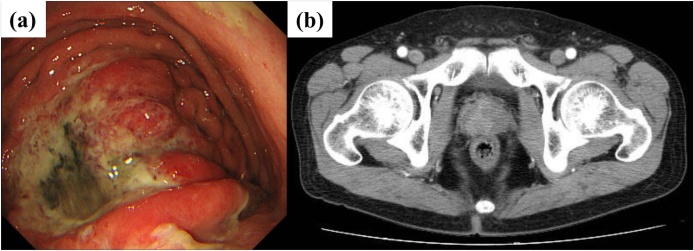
Preoperative image findings. (a) Gastroscopy revealed a type 3 tumor on the greater curvature of the stomach. (b) Prostate cancer was suspected to spread out of the capsule by contrast-enhanced CT.
